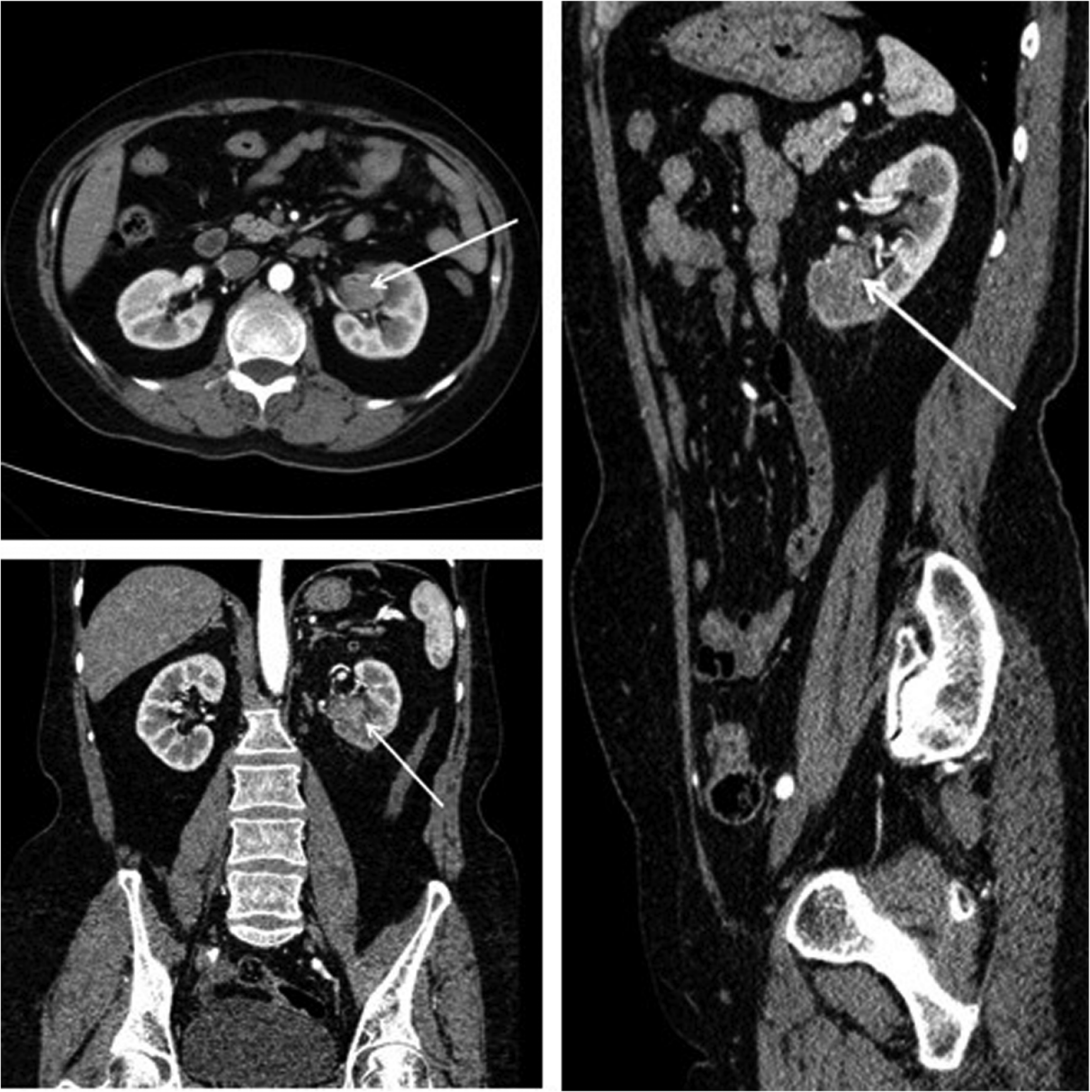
Determination of the tumor location by CT. Arrows indicate an upper urinary tract urothelial carcinoma
